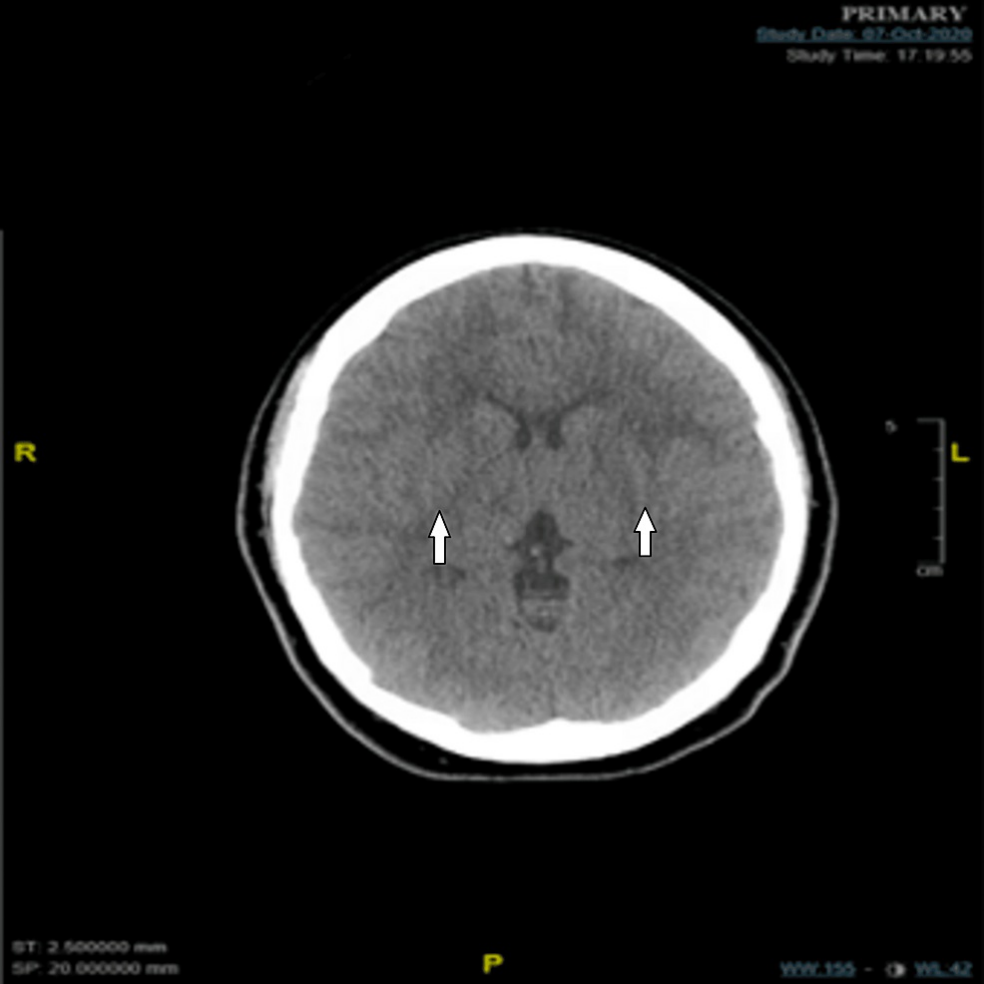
Computerized tomography (CT) of the head without contrast White arrows showing mild bilateral white matter hypoattenuation
